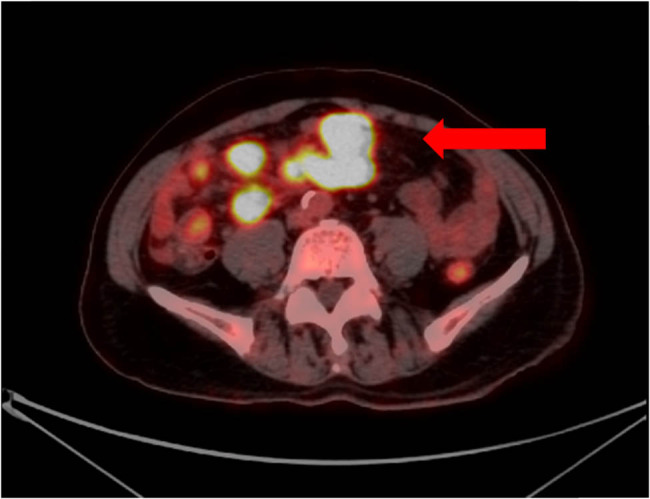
18F-FDG PET/CT image revealed that the intestinal wall of some small intestine in the lower abdomen was significantly thickened segmentally, multiple lymph nodes were found in the retroperitoneal space, some of them were fused into clusters, and the glucose metabolism was abnormally increased (arrowhead).
